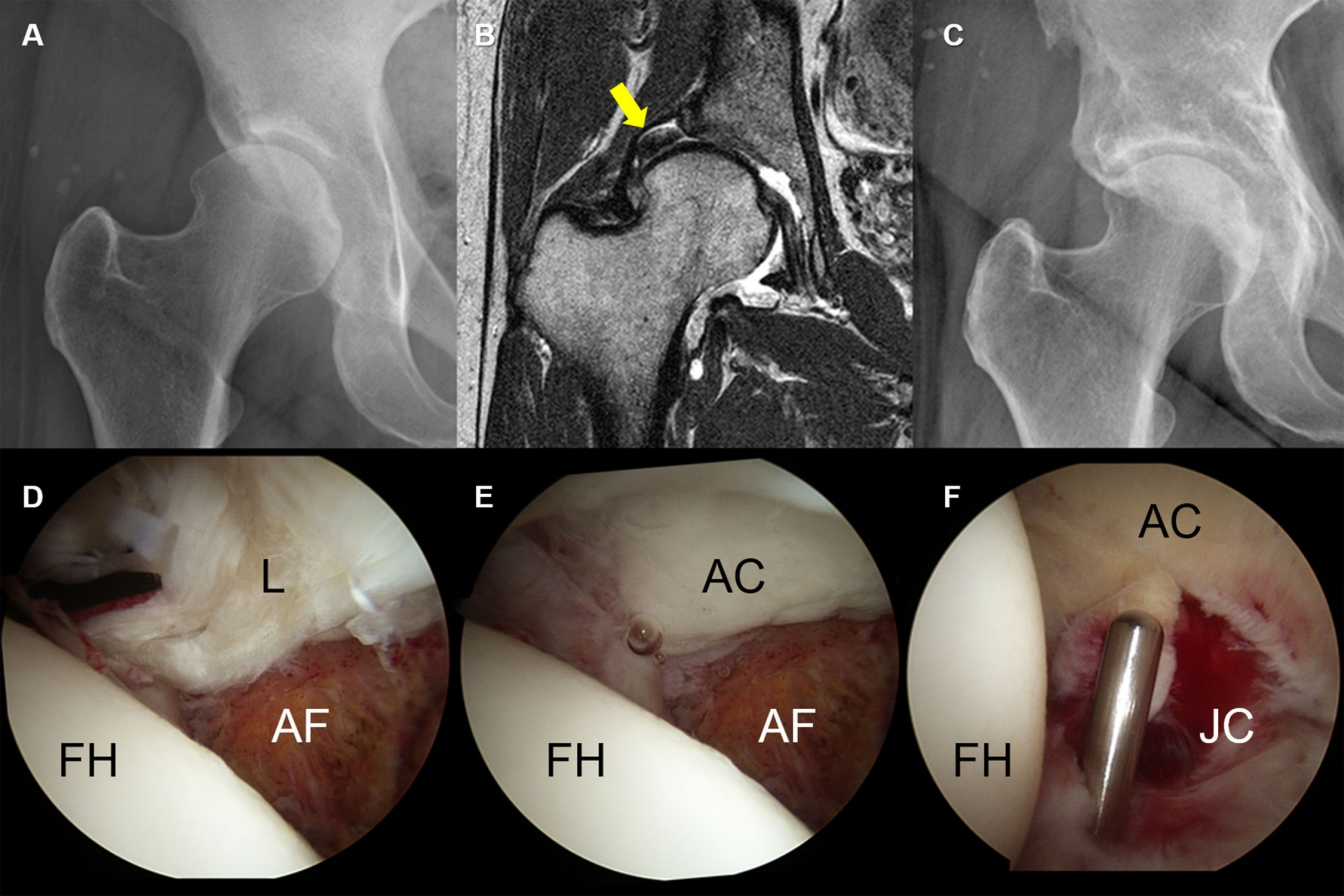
A grade 4 labral injury in a 45-year-old woman. (A) Simple X-ray showing joint space narrowing. (B). Coronal MRI image showing complete lateral displacement of the labrum (arrow). The patient underwent arthroscopy combined with PAO. (C) Follow-up X-ray at postoperative 3 years. (D, E) Arthroscopy showing labral displacement was shown as a bucket-handle tear. (F) Partial resection to remove an unstable degenerative labral tear. (L, labrum; FH, femoral head; AF, acetabular fossa; AC, acetabular cartilage; JC, joint capsule).
